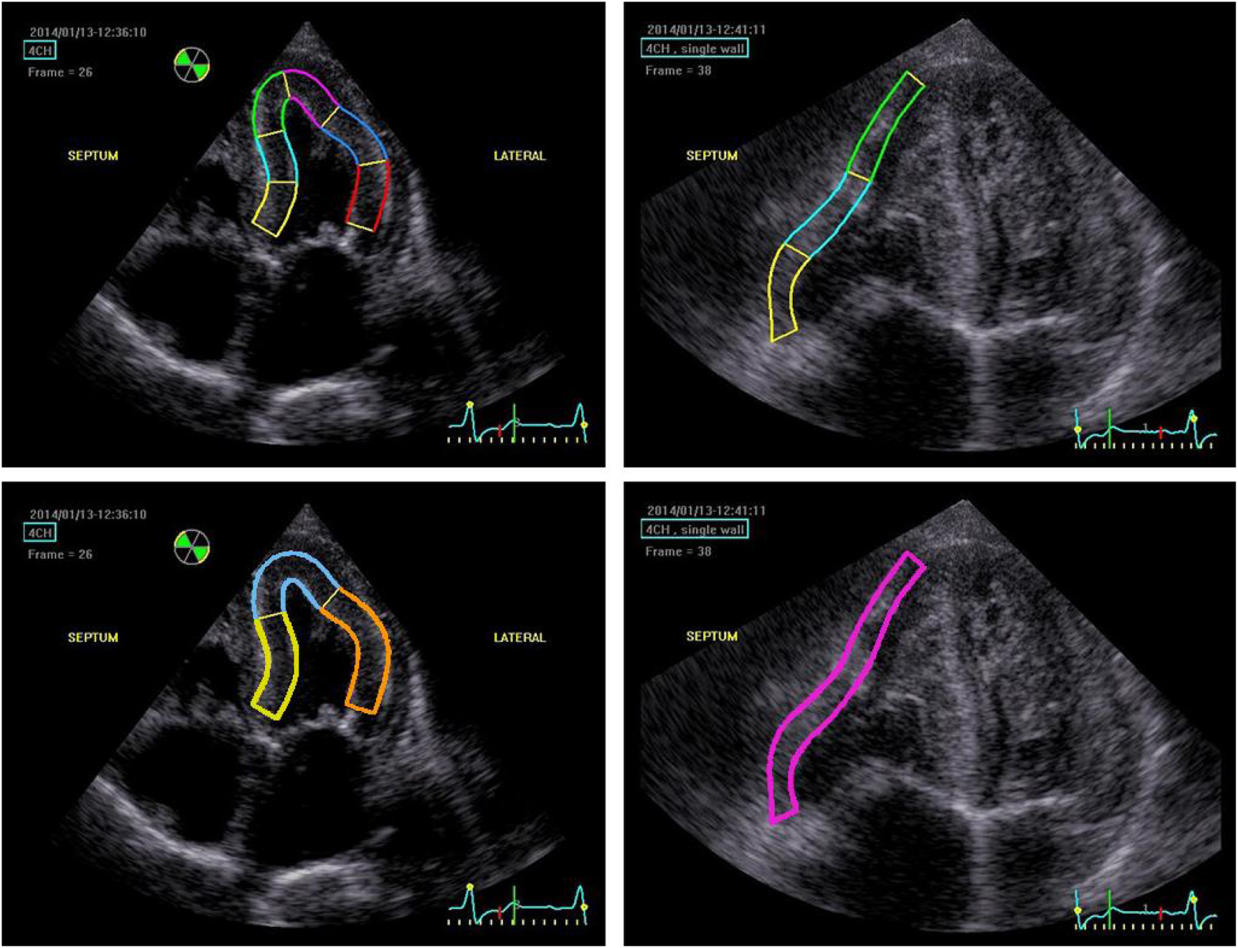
Images of echocardiographic speckle tracking strain analysis in apical four-chamber view projections. Top panels show full wall regions of interest (ROIs) mappings for left ventricular global longitudinal strain (LV) (left) and right ventricular free wall strain (RV free wall) (right). Bottom panels show how different echocardiographic ROIs match the DPD scintigraphic volumes of interest (VOIs) described in Fig. 2
